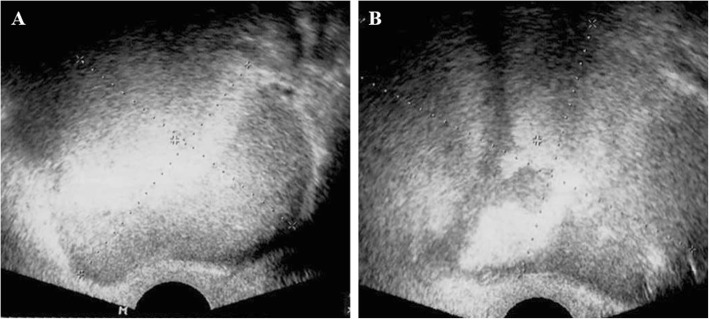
Transvaginal ultrasonography showing mixed echogenic masses in both right (a) and left (b) adnexa
